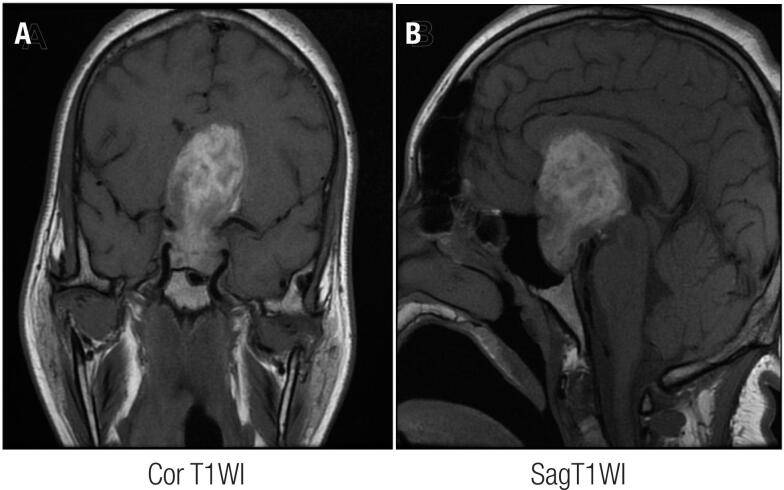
Coronal T1 (A) and sagittal T1-weighted imaging (B) sequences of the magnetic resonance imaging of a 16-year-old patient performed three days after the pituitary apoplexy event and showing a mass in the sellar region with important suprasellar and infrasellar extension, presenting hyperintense areas.
